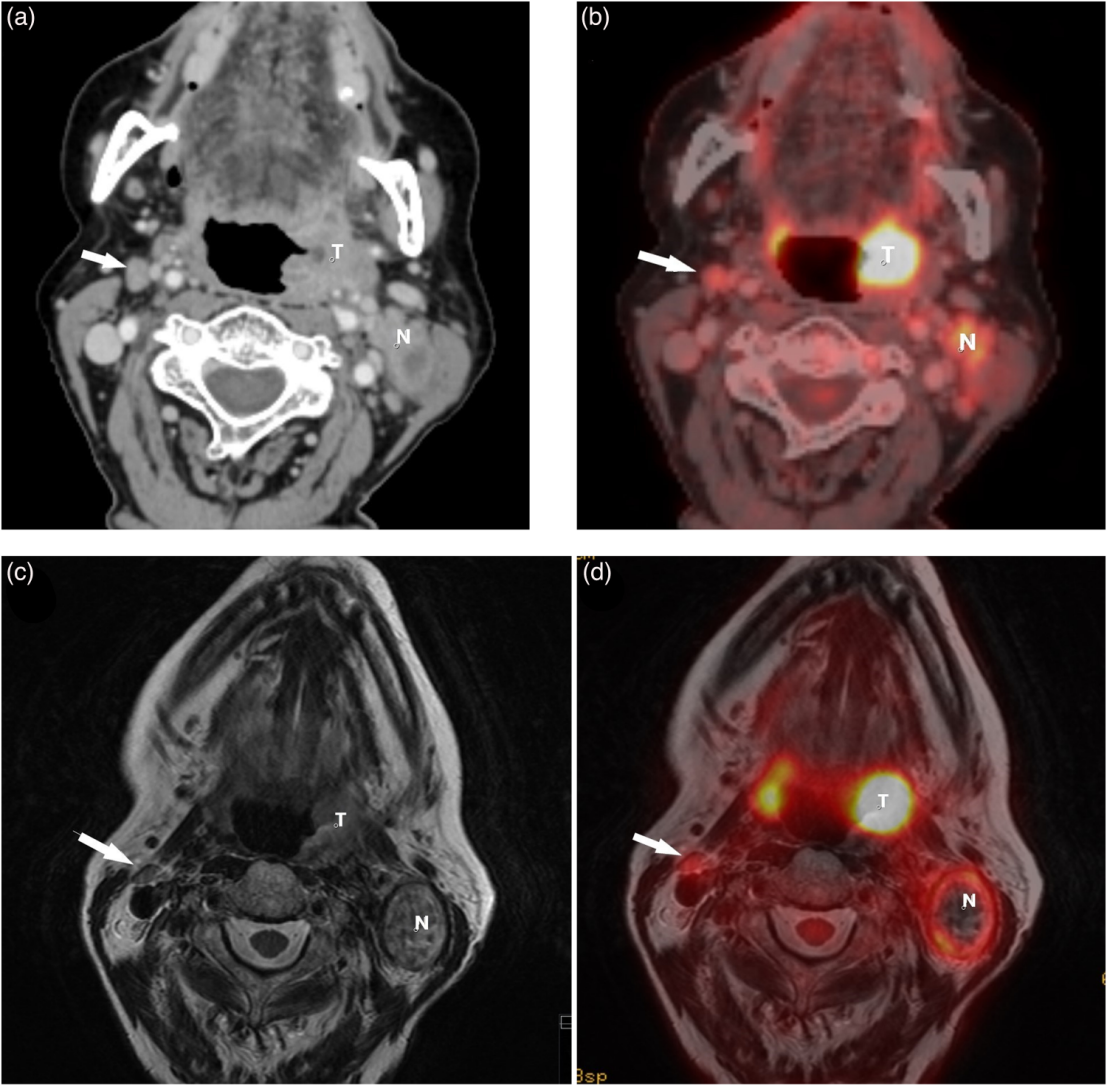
A patient with a SCC in the left tonsil and ipsilateral metastasis. The right-sided node (arrow) was judged as metastatic on PET/CT (false positive) but not on PET/MRI (true negative). US-FNAC of the node revealed no malignant cells, and the node was at considered reactive at the MDC. Arrow = contralateral reactive node. (a) CT scan reveals slightly enlarged, rounded right-sided node (arrow). (b) A FDG-PET/CT hybrid image shows the node (arrow) has an elevated FDG-tracer uptake (SUV_max_ 5.4). (c) On T2W MRI, the node (arrow) has a normal fusiform appearance. (d) FDG-PET/MRI hybrid image demonstrates mild FDG-tracer uptake (SUV_max_ 4.5) in the node (arrow). CT, computed tomography; MDC, multidisciplinary conference; MRI, magnetic resonance imaging; N, metastatic ipsilateral node; PET, positron emission tomography; SCC, squamous cell carcinoma; T, tumor; T2W, T2-weighted; US-FNAC, ultrasound-guided fine-needle aspiration cytology.
